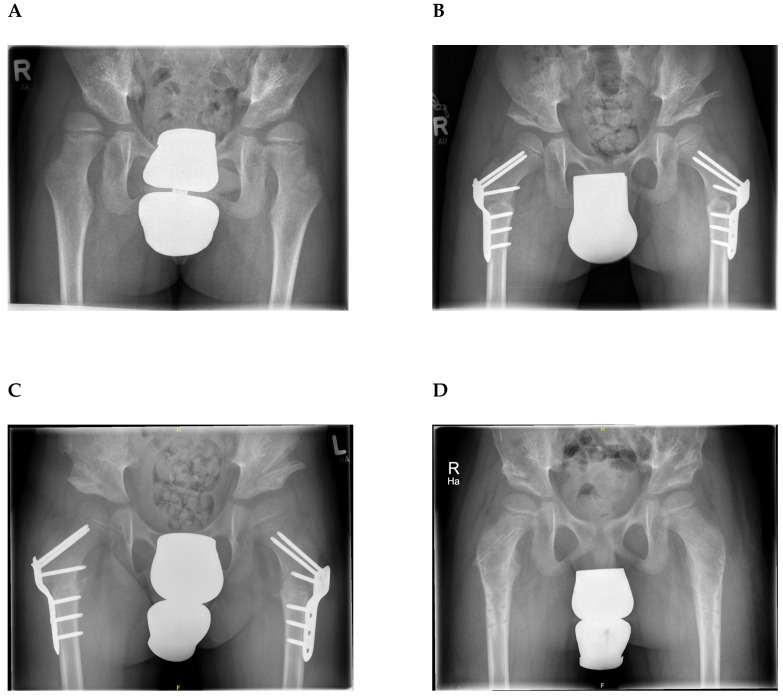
(A) Preoperative anteroposterior radiograph of the pelvis of a 6-year-old boy with hip dislocation and acetabular dysplasia on both sides. (B) Postoperative radiograph one week after bilateral proximal femoral varus derotation osteotomy and Dega osteotomy using Tutobone^®^ shows a significant improvement of acetabular inclination and coverage of the femoral head on both sides. (C) The position of the Tutobone^®^ block as well as acetabular indices remained stable after 6 weeks of immobilization (D) The correction of acetabular dysplasia remained stable at the 1-year follow-up examination and the Tutobone^®^ block was incorporated (Goldberg score 6 on the right and 7 on the left side). The femoral plates had been removed one year after surgery.
